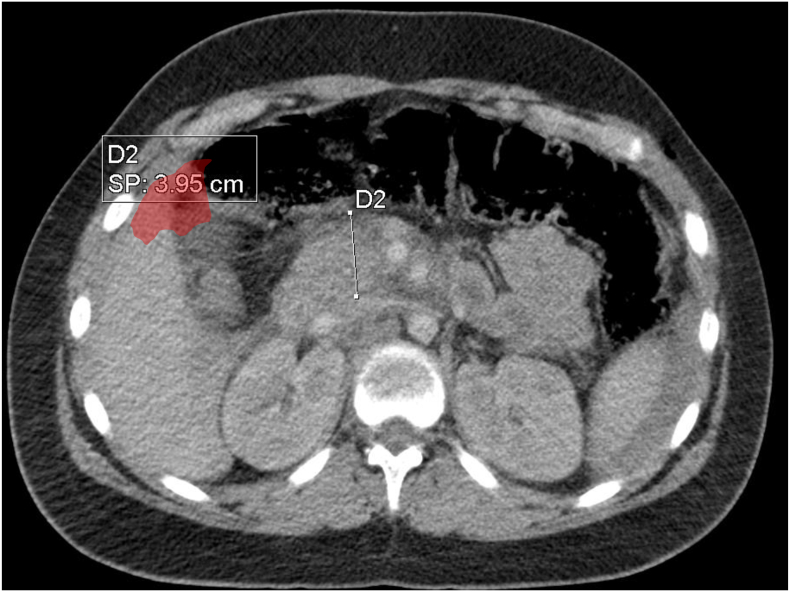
Axial computed tomographic section demonstrating swollen pancreas in its cephalic portion, measuring 39 mm in maximal diameter, corresponding on contusion grade III.
